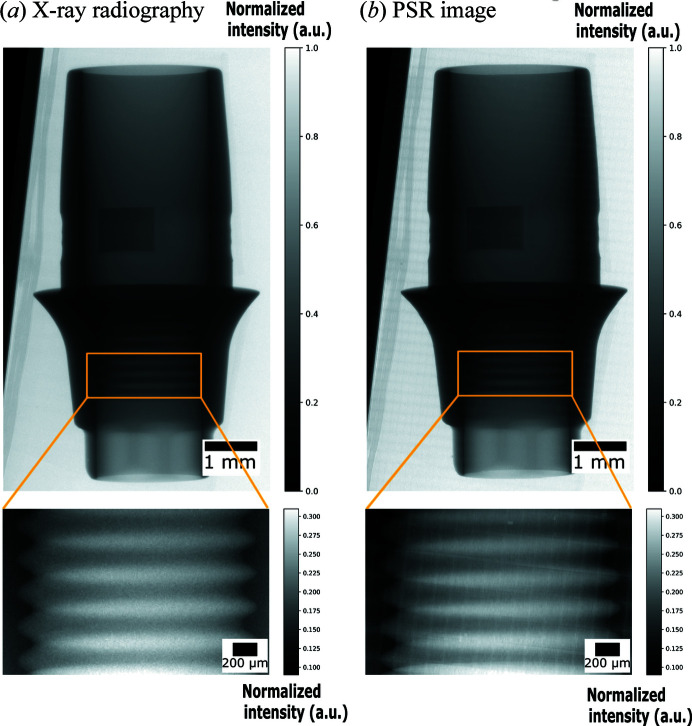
Application of the PSR-STHXM for investigating biomedical implants with a zoomed-in view of the internal thread fabricated via selective laser melting. (*a*) Conventional X-ray radiography image (pixel size 8.03 µm × 8.03 µm). (*b*) PSR-STHXM image (pixel size 300 nm × 8.03 µm).
